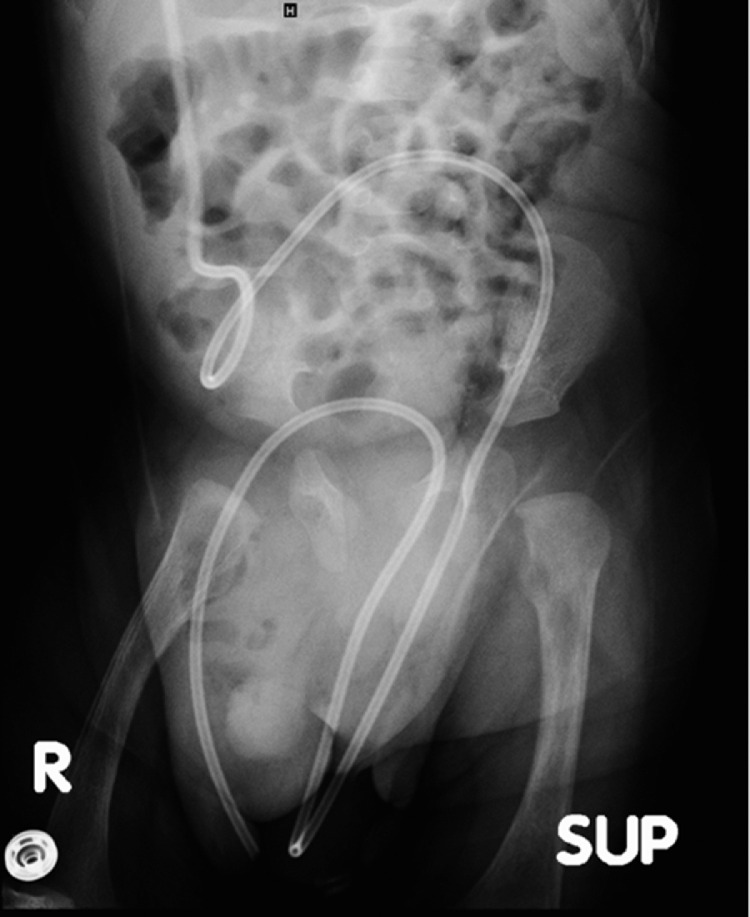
Follow-up abdomen radiograph showing distal tip of VP shunt projects over right scrotal sac.
